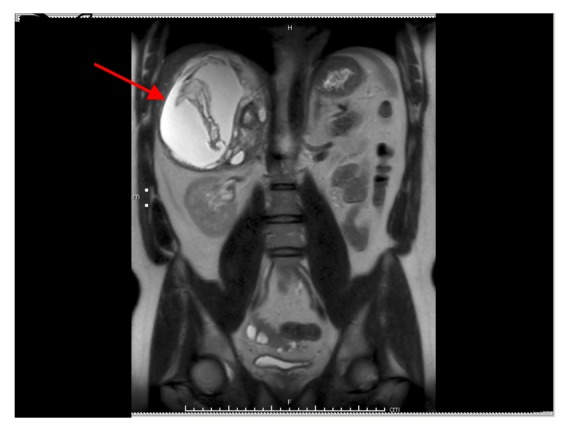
MRI abdomen shows 11 x 12 x 14 cm mixed cystic and solid mass centered in the right adrenal gland.
